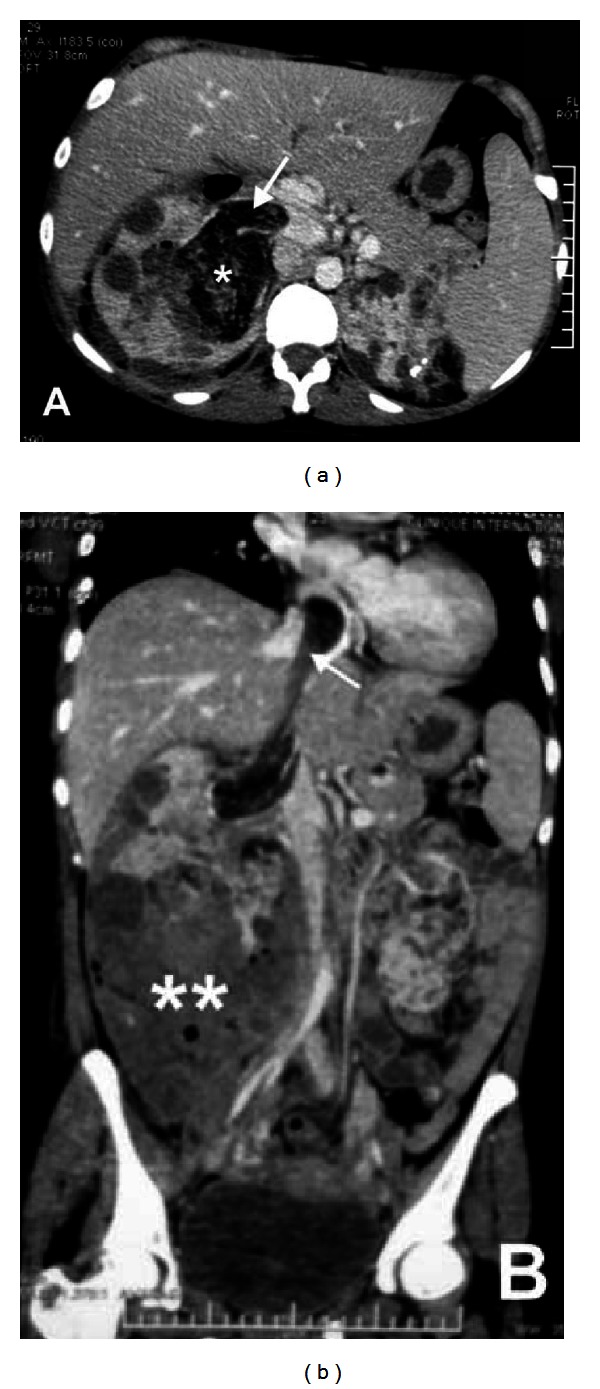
(a) Coronal CT scan showing renal AML (∗) with inferior vena cava thrombus (arrow). (b) The thrombus extends up to right atrium. Note the presence of a large perirenal infected hematoma due to an old bleeding from lower pole AML (∗∗).
